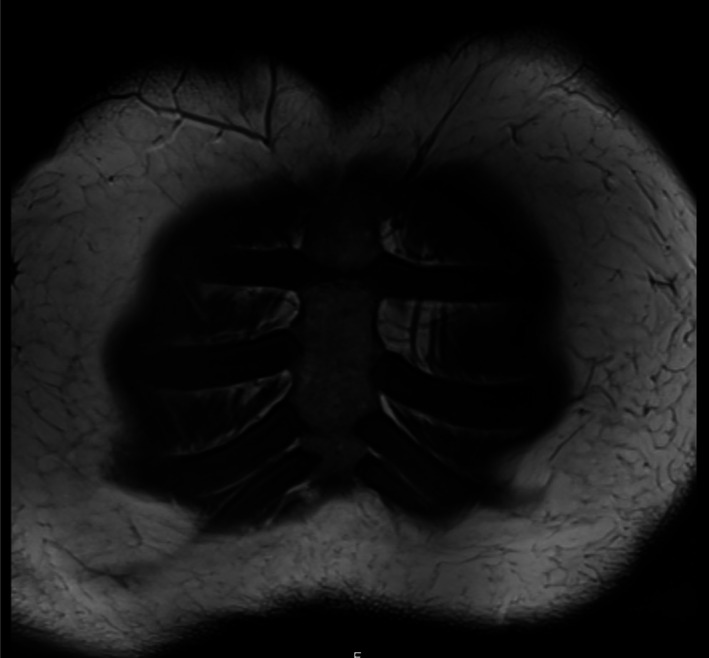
Sagittal T1‐weighted magnetic resonance imaging (MRI) of the sternum. Structural erosions are visible at the margins of the chondrosternal joints. These chronic inflammatory changes support the diagnosis of axial spondyloarthritis in the context of CC. Note the absence of significant bone marrow edema or surrounding soft tissue swelling.
